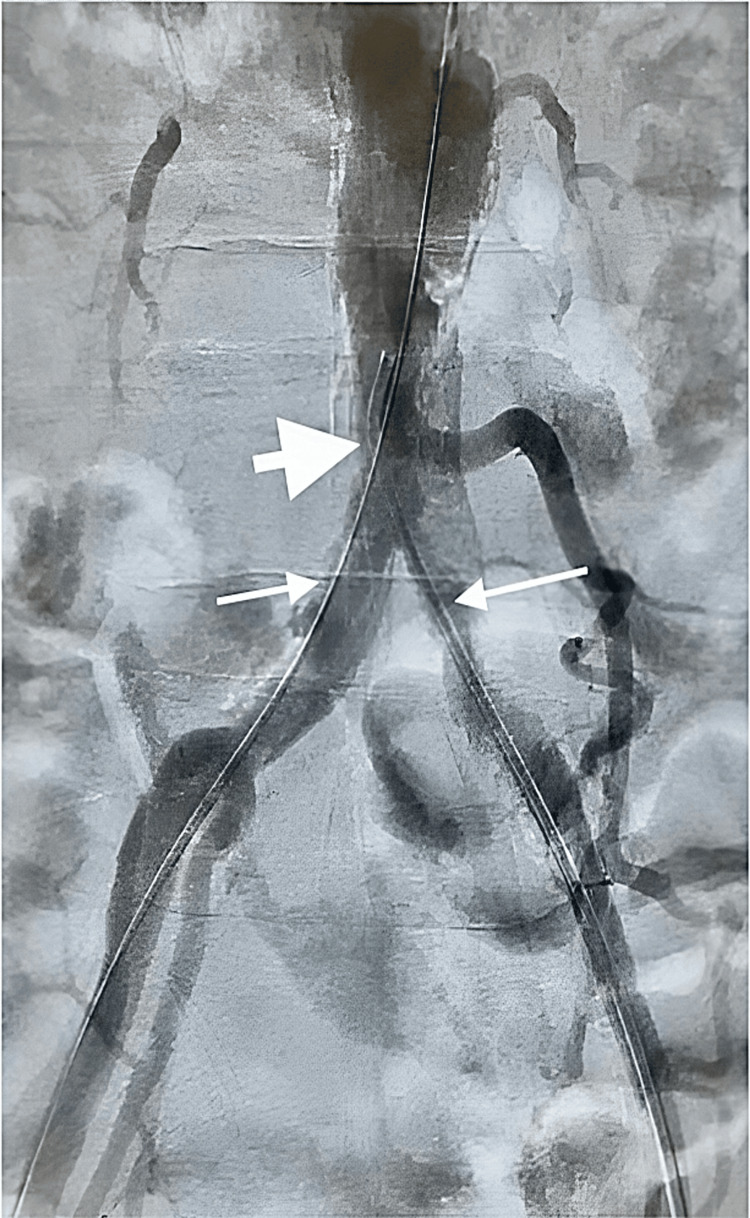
Post lithotripsy balloon angioplasty computed tomography (CT) angiography demonstrating marked improvement in distal aorta and bilateral external iliac artery blood flow.
